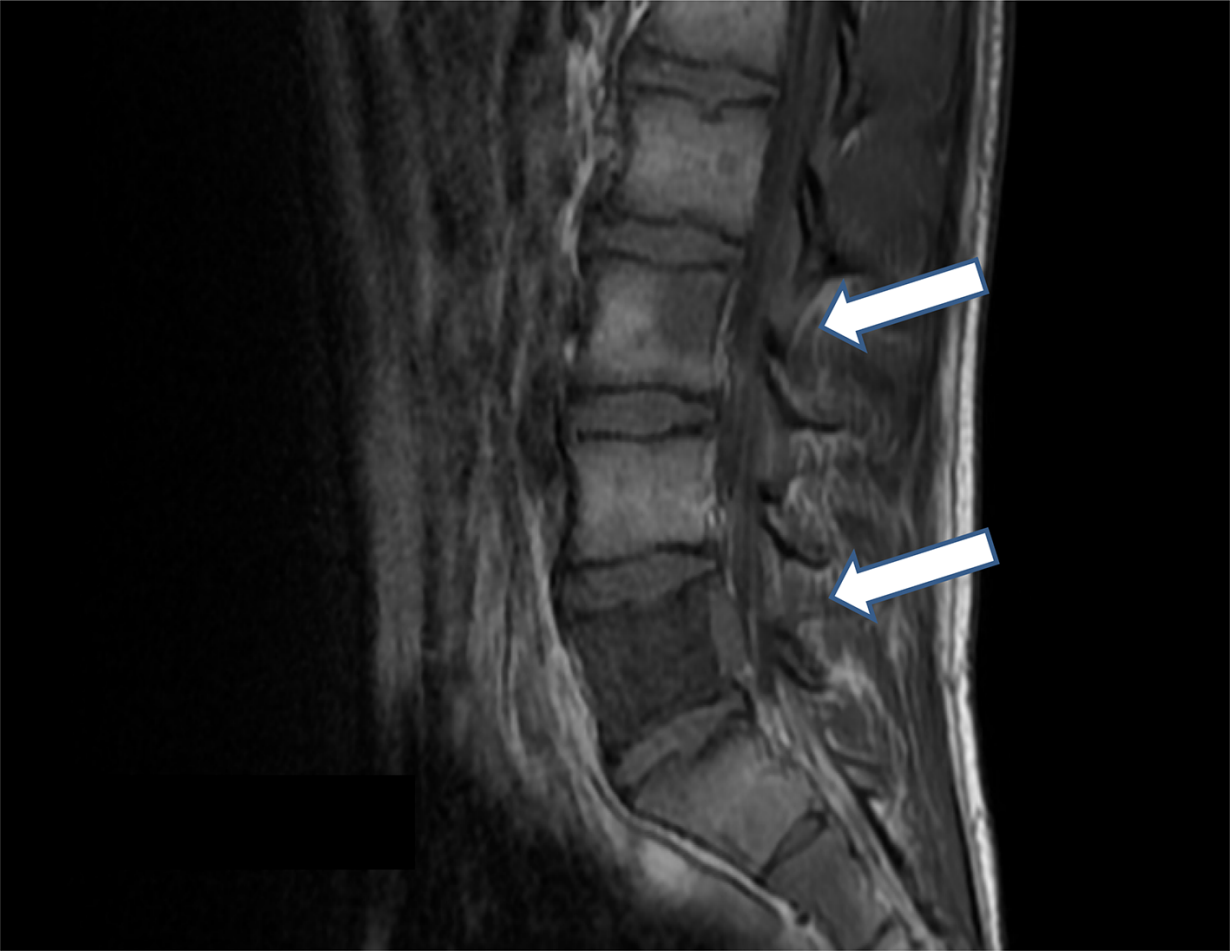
Magnetic resonance imaging showed osteolytic lesions in L3 and L5, suggesting bone metastases.
